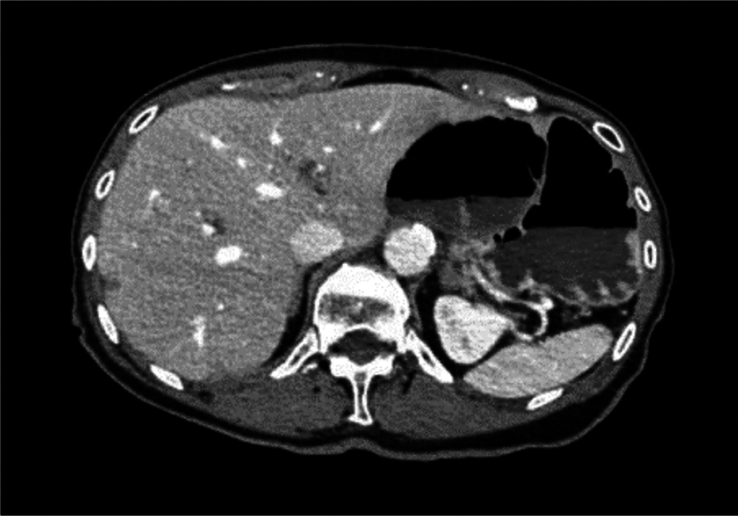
Contrast-enhanced computed tomography scan in the portal-venous phase of the upper abdomen showing the dilated colon next to the stomach.
